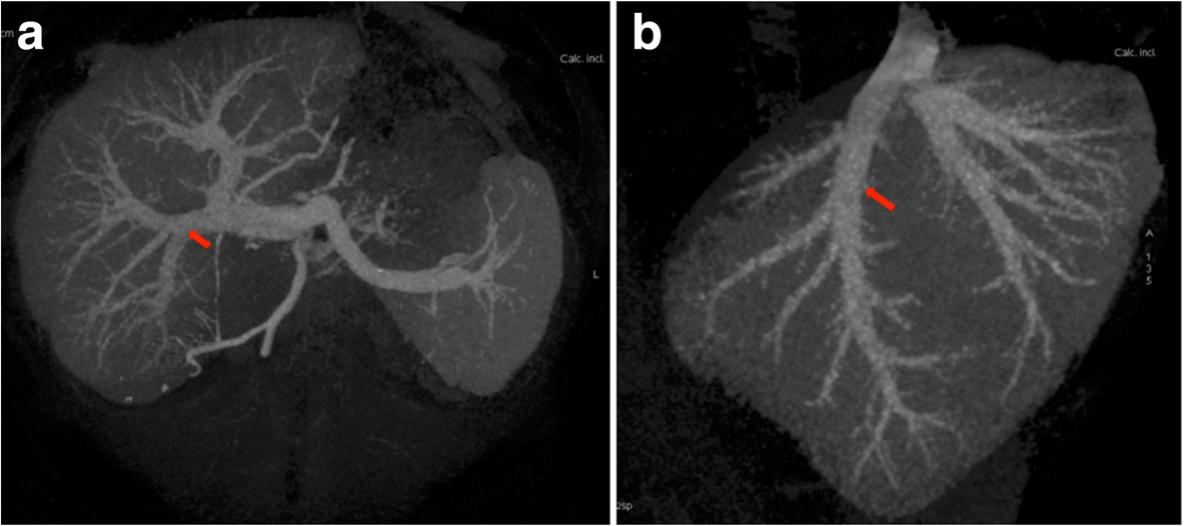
Preoperative liver triple phase CT-scan showed normal appearance of right hepatic vein (a) and right portal vein (b)
